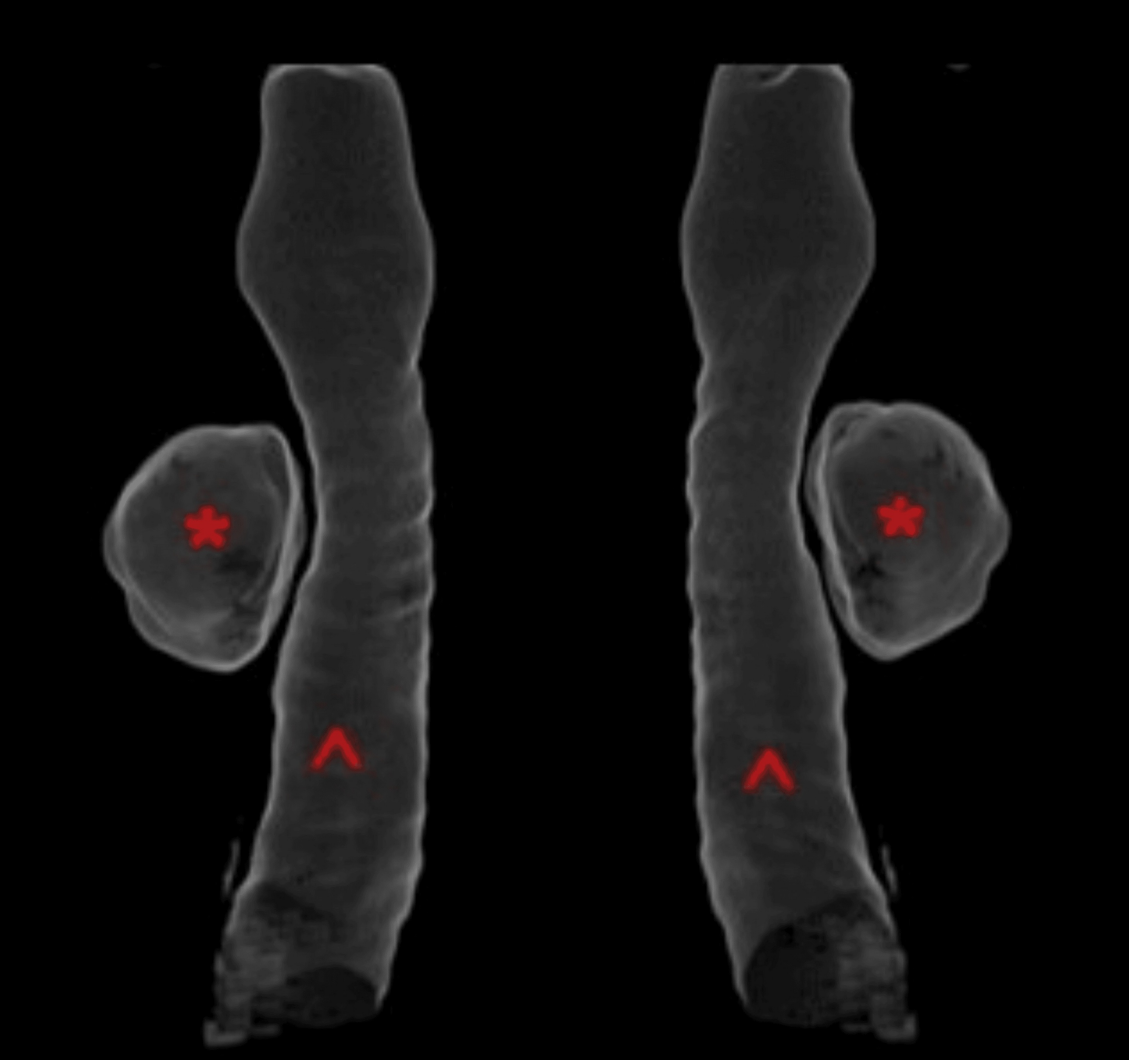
Three-dimensional reconstruction of the trachea derived from thoracic CT scan (1.25-mm slice) Right-sided tracheal diverticulum (*), without detectable connection to the trachea (^)
